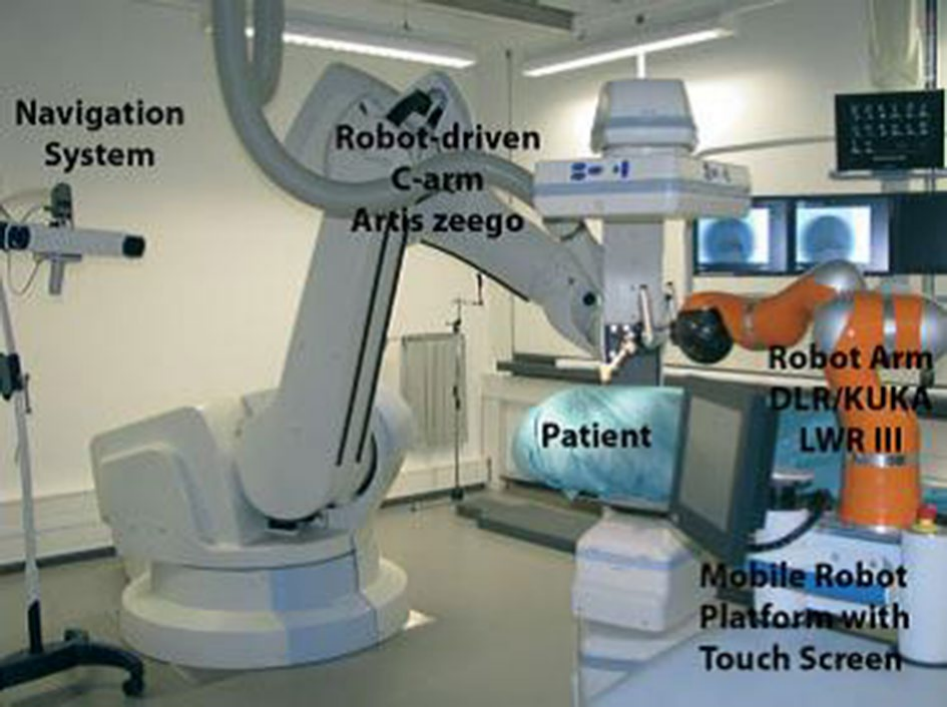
System for CT-guided robotically‐assisted interventions. Reprinted with permission from [58]
